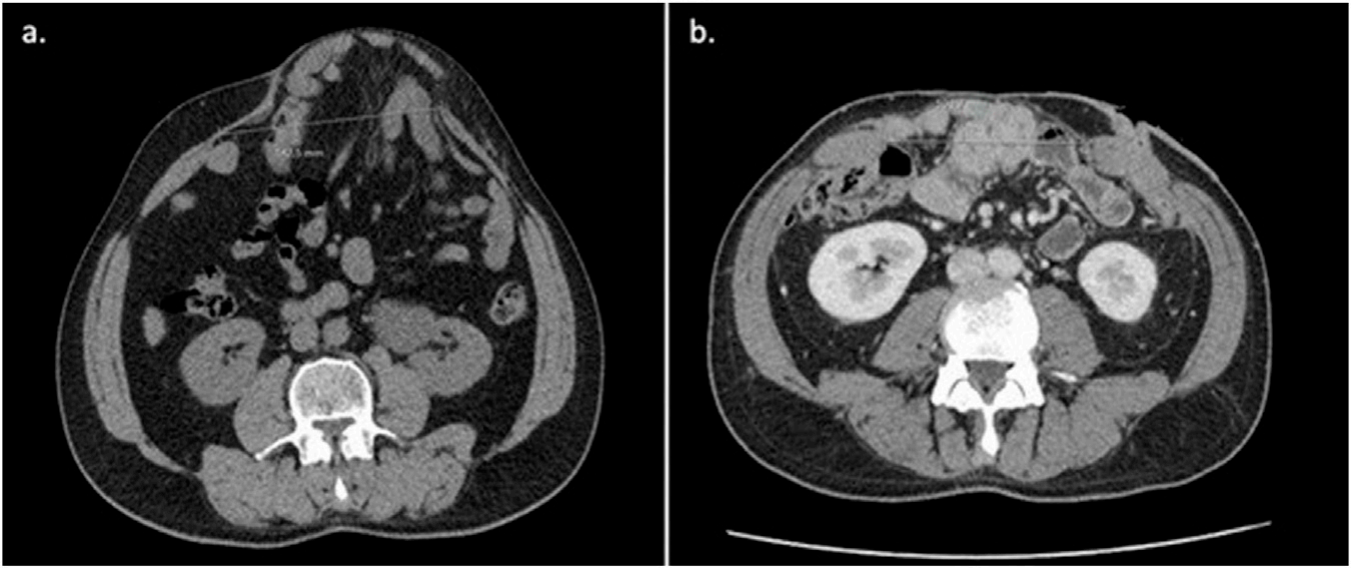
Examples of lateral muscle retraction on CT patients submitted to BTA and IFT. Lateral muscle retraction is indirectly estimated by observing the relationship between an increase in muscle thickness and a corresponding decrease in muscle length. The decrease in muscle length is typically associated with increased hernia width.
